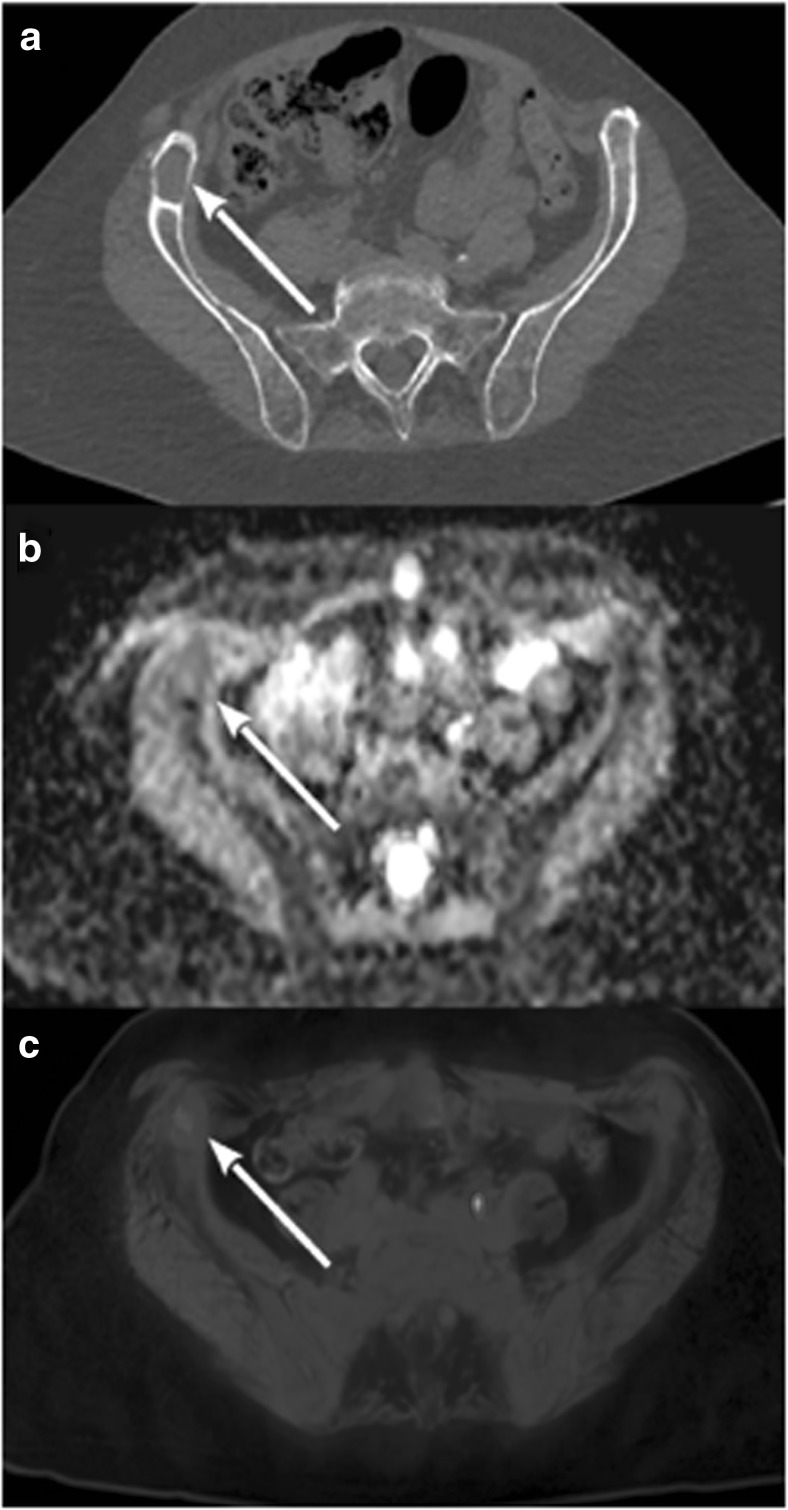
a WB-LDCT showing lytic bone lesion with positive densitometry of 53 HU. b ADC map with ADC value of the lesion of 876 × 10^−6^ mm^2^/s. c Fused PET/RMI image showing hypermetabolism of the lesion with SUVmax of 3.9
